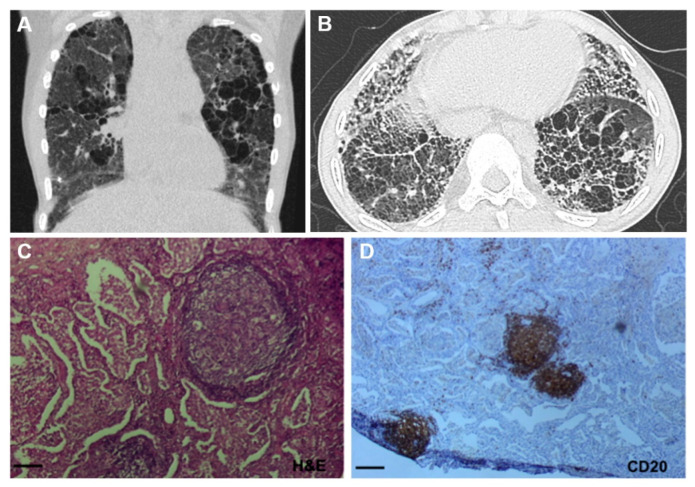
Representative Lung Imaging and Pathology in SAVI. (A,B) Coronal and axial image of a chest computed tomography (CT) scan from a SAVI patient showing interstitial lung disease with ground glass opacities, cysts, and septal wall thickening. (C,D) Lung tissue section biopsy from a SAVI patient showing lymphoid infiltrate consisting mainly of CD20+ cells. Original magnification: ×40 (C; scale bars, 100 µm), ×10 (D; scale bars, 400 µm) Adapted from Jeremiah et al., J Clin Invest, 2014 and Frémond et al., J Allergy Clin Immunol Pract, 2021.
